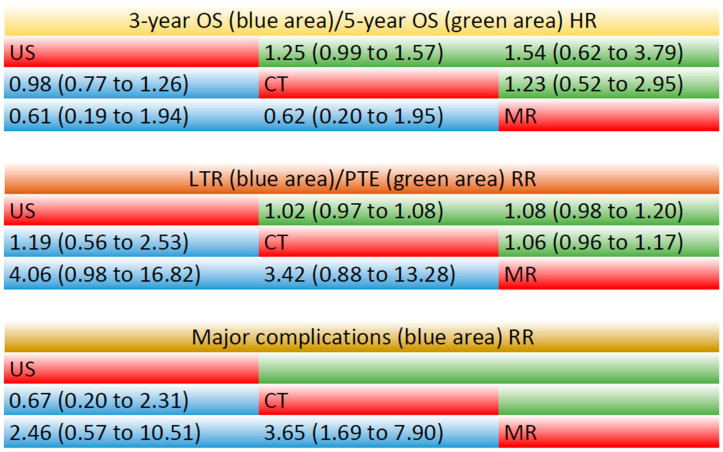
The league table shows hazard ratios (HRs) or relative ratios (RRs) for pairwise comparisons of the 3-year OS, 5-year OS, LTR, PTE, and major complications among the image modalities. The comparisons should be read from left to right. HRs (95%CI) or RRs (95%CI) for the comparisons are in the cells shared by the column-defining and row-defining interventions. CT, computed tomography; MR, magnetic resonance; US, ultrasound.
